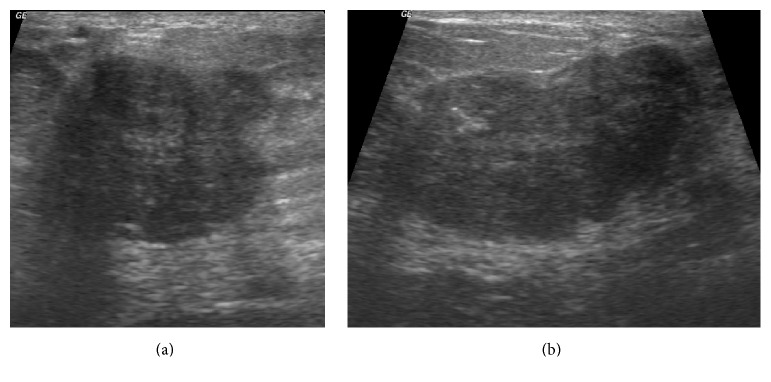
Transverse (a) and longitudinal (b) ultrasonography images showing a solid hypoechoic and heterogeneous mass with microlobulated contours, measuring 3.6 cm × 2.9 cm × 2.3 cm, with long transversal axis of the external superior quadrant of the right breast (collection of the Department of Imaging, the University Teaching Hospital of Lomé).
